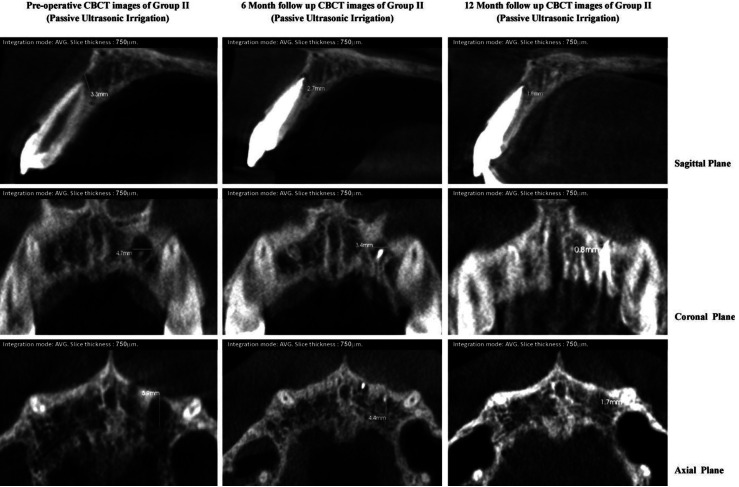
CBCT images of group II (passive ultrasonic irrigation) showing pre-operative and post-operative dimension of periapical lesion in all three planes.
